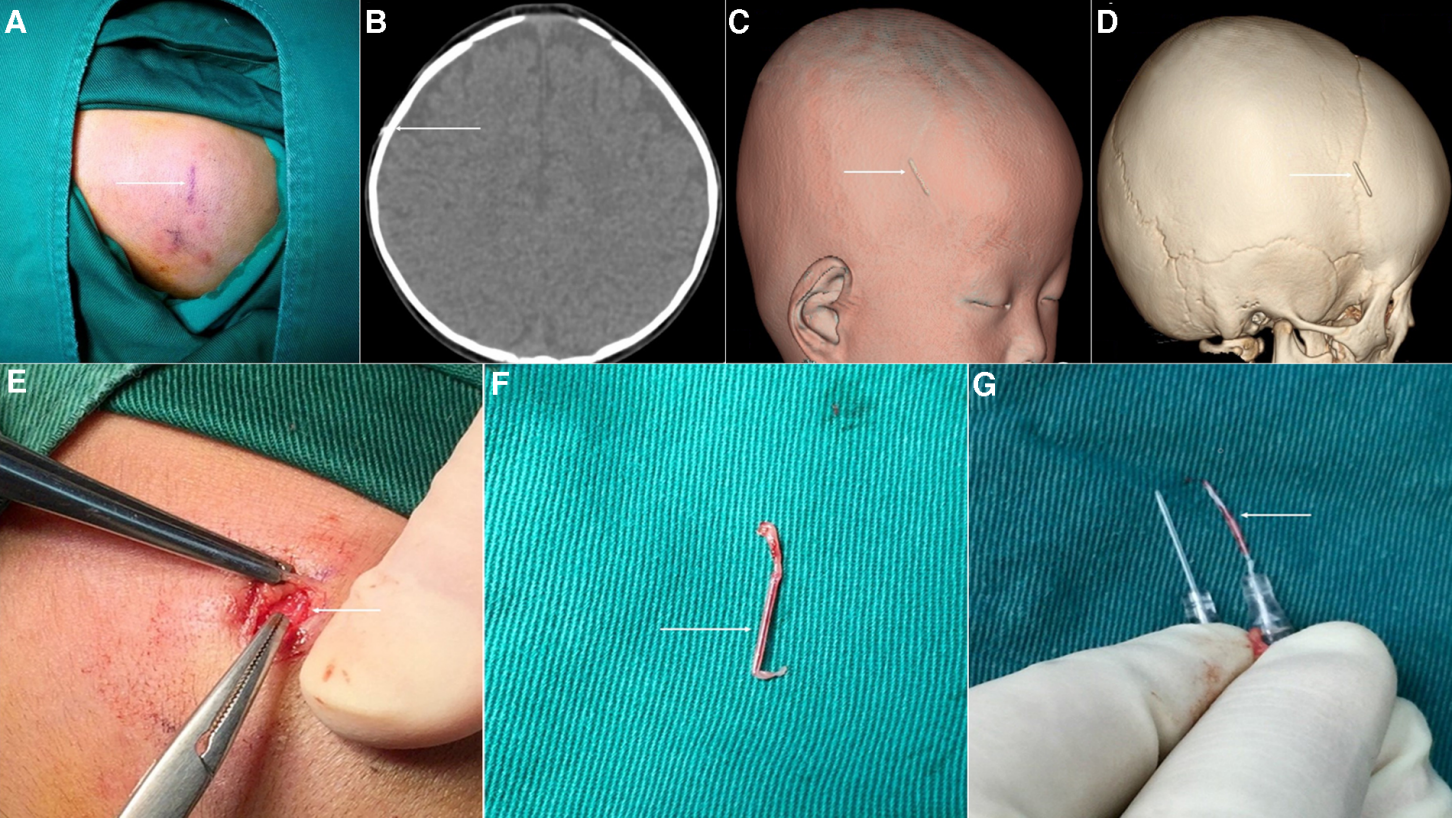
Surgical removal of the indwelling needle from the broken scalp vein. (A) The local puncture area of the skin near the superficial forehead vein is red and swollen with cord-like changes. (B) The broken vein indwelling needle is seen as high density on ordinary CT scan. (C,D) 3D-CT imaging clearly shows the specific location of the fracture, as well as the surrounding bone markers. (E) Blood vessels are seen completely wrapped around the broken indwelling needle. (F) The broken catheter was removed successfully during the operation. (G) The broken end coincides with the residual needle stem, which is consistent with the length of the normal indwelling needle.
